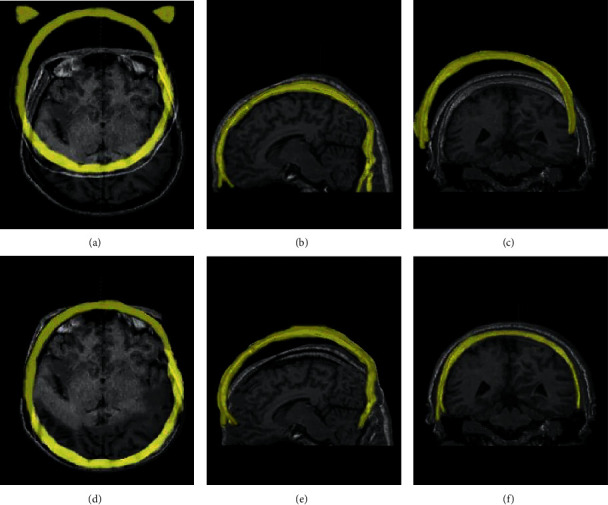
MRI images under the three-dimensional mark point positioning algorithm.
